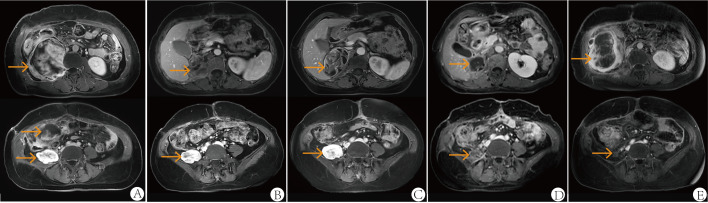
Abdominal MRI at first relapse in October 2021 (A), one month after the second surgery in April 2022 (B), after two cycles of second-line therapy (gemcitabine plus albumin paclitaxel) in June 2022 (C), after six cycles of third-line therapy (eribulin plus sintilimab, combined with radiotherapy) in December 2022 (D) and at follow-up in June 2023 (E). All images were acquired with T1-weighted sequences.
